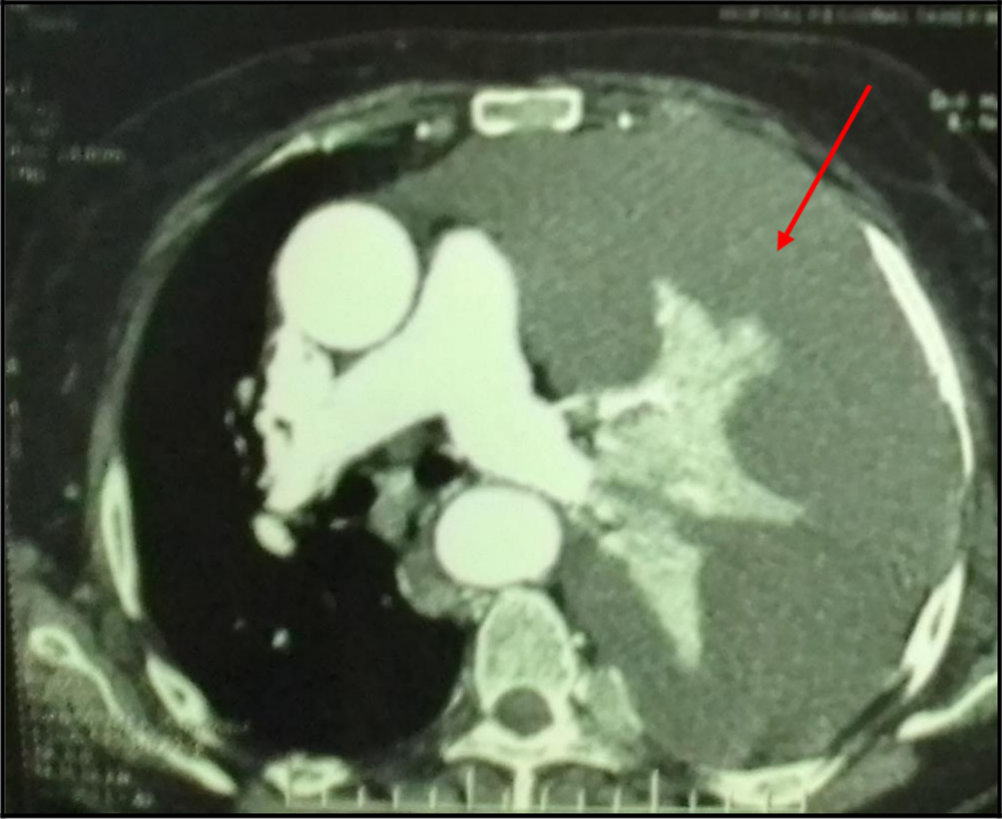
A mediastinal chest CT section revealing a massive pleural effusion in the left side (red arrows).
